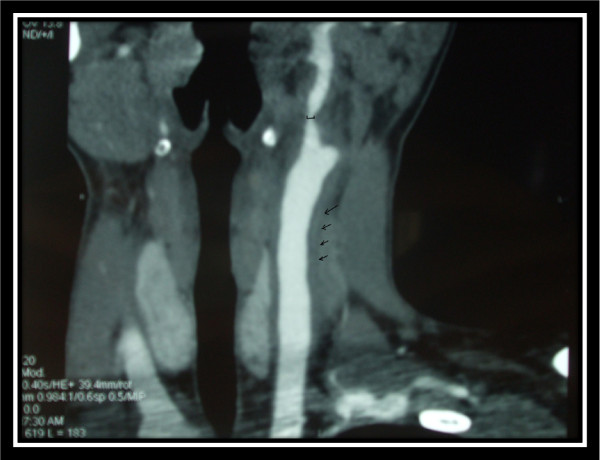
Cervical CT angiography shows regular thickening of the left common carotid artery and left external carotid stenosis.
